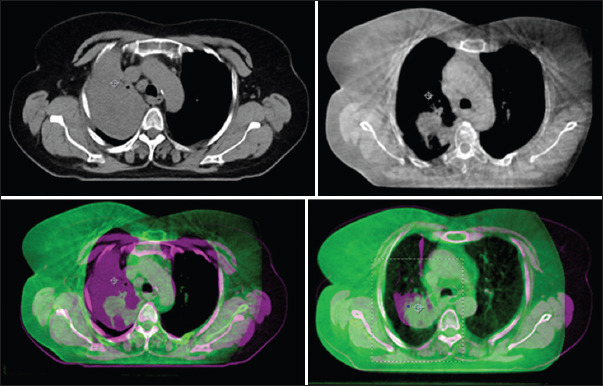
A 55-year-old woman diagnosed with non-small-cell lung cancer cT3 N2 M0 was treated with concurrent chemotherapy and radiation. This patient underwent intensity-modulated radiotherapy (RT) with image-guided RT (daily cone-beam computed tomography [CBCT]). Initial atelectasis improved until total resolution after 20 Gy. Adaptive RT (ART) was implemented by the treating physician to spare as much lung tissue as possible while keeping the tumor covered. The lower panel shows reference CBCT pre-ART (red) and post-ART (green).
